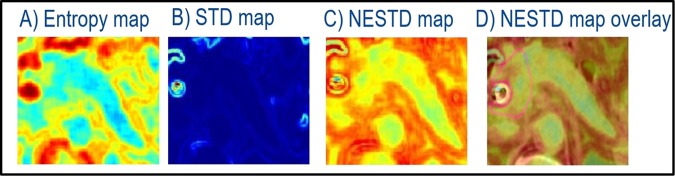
An example of NESTD map generation. a entropy map, b STD map, c the resultant NESTD map, and d the NESTD map overlaid on the original CT image
